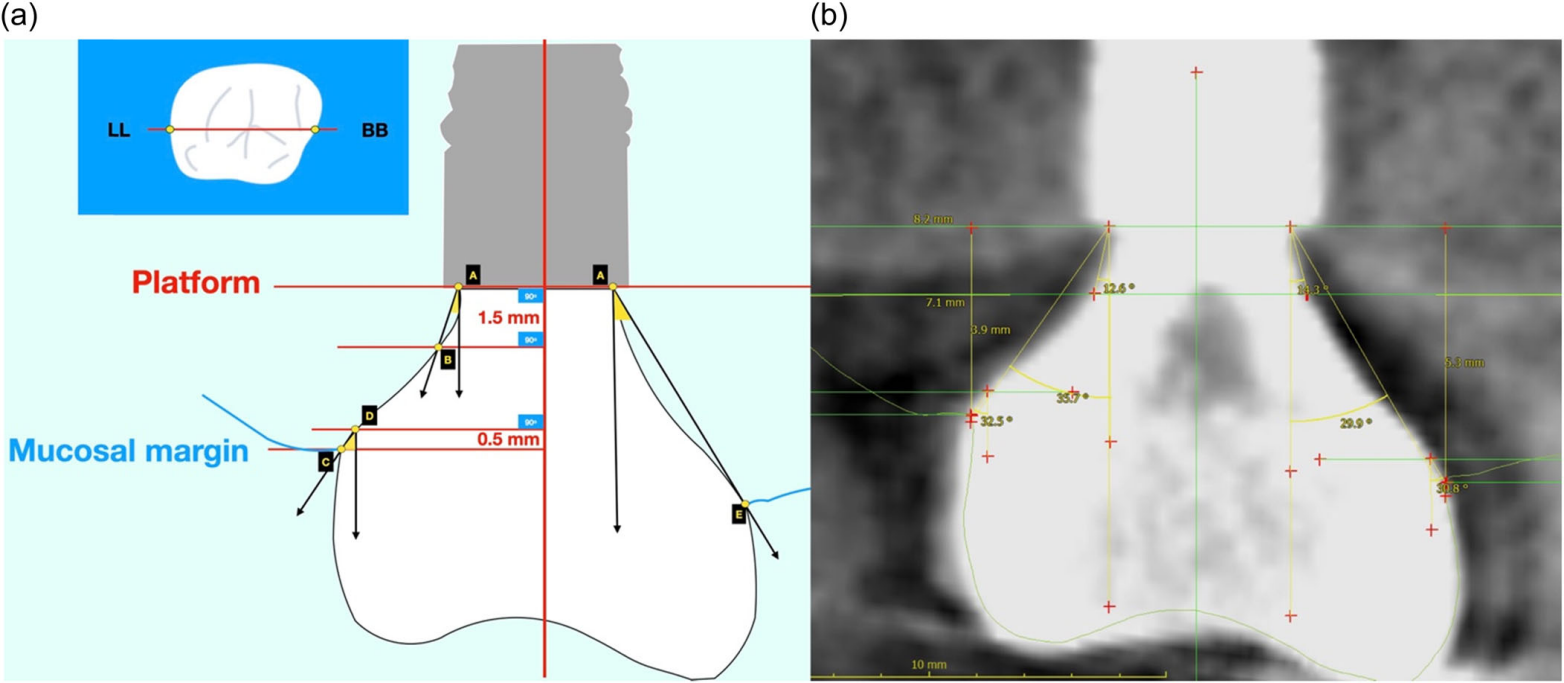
(a) Buccal—Palatal slice and definition of 4 horizontal planes perpendicular to implant axis, 5‐points (A‐E) and the respective 3 angles measured on each side (six sites per implant). (b) sample of actual radiographic measurement screen.
